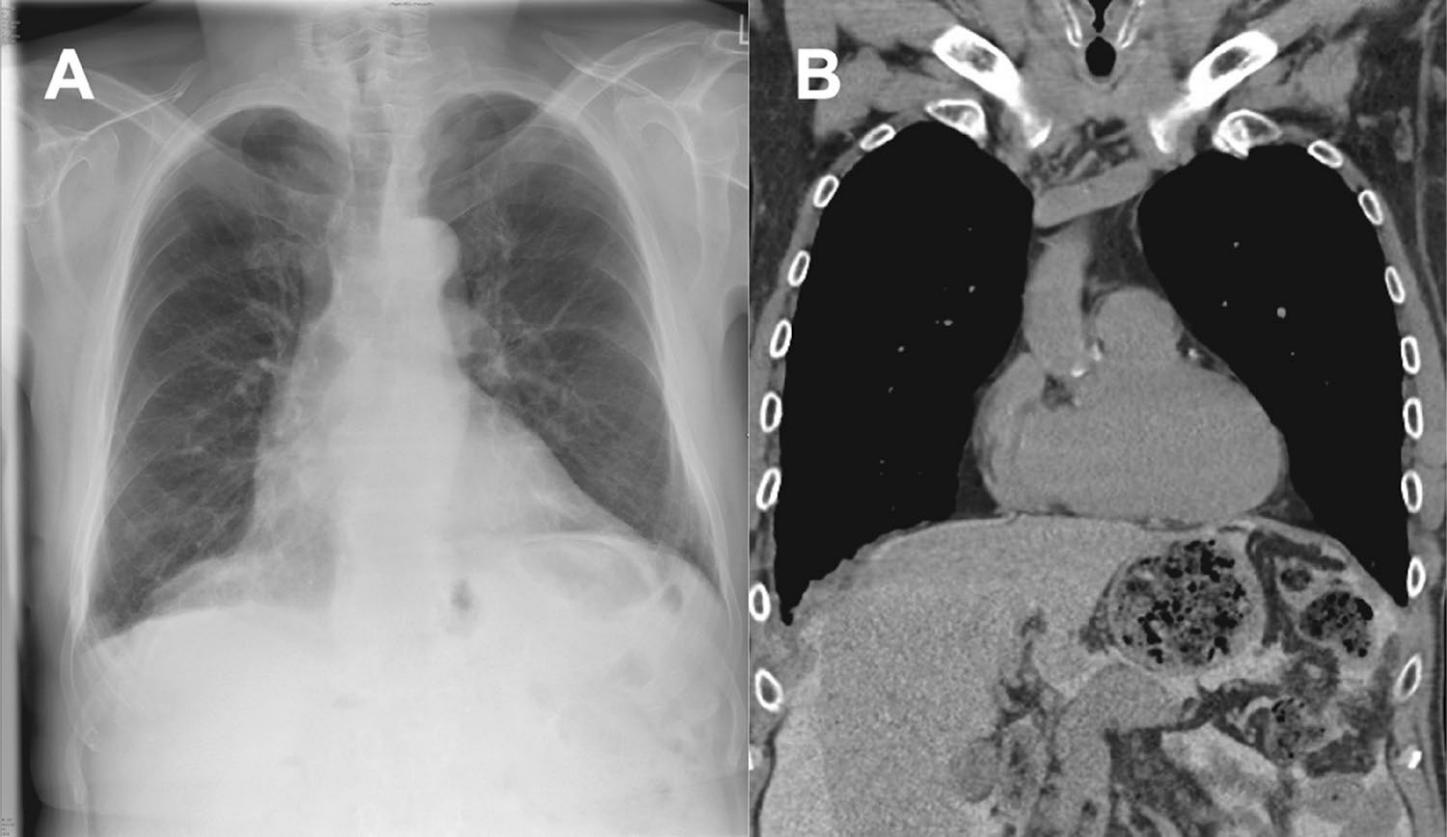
Radiologic appearance after mesh implantation in a 74-year old male peritoneal dialysis patient. A Chest x-ray 3 months after mesh implantation on the right diaphragm. B Computed tomography of the thorax 12 months after mesh implantation on the right diaphragm
